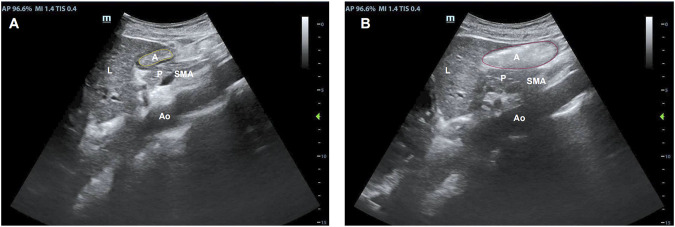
Sonographic identification of the gastric antrum with the aid of anatomical landmarks: A, the gastric antral cross-sectional area; Ao, the abdominal aorta; L, the left lobe of the liver; P, the inferior pancreas; and SAM, the superior mesenteric artery. The gastric antrum cross-sectional area after (A) fasting and (B) immediately after a meal.
